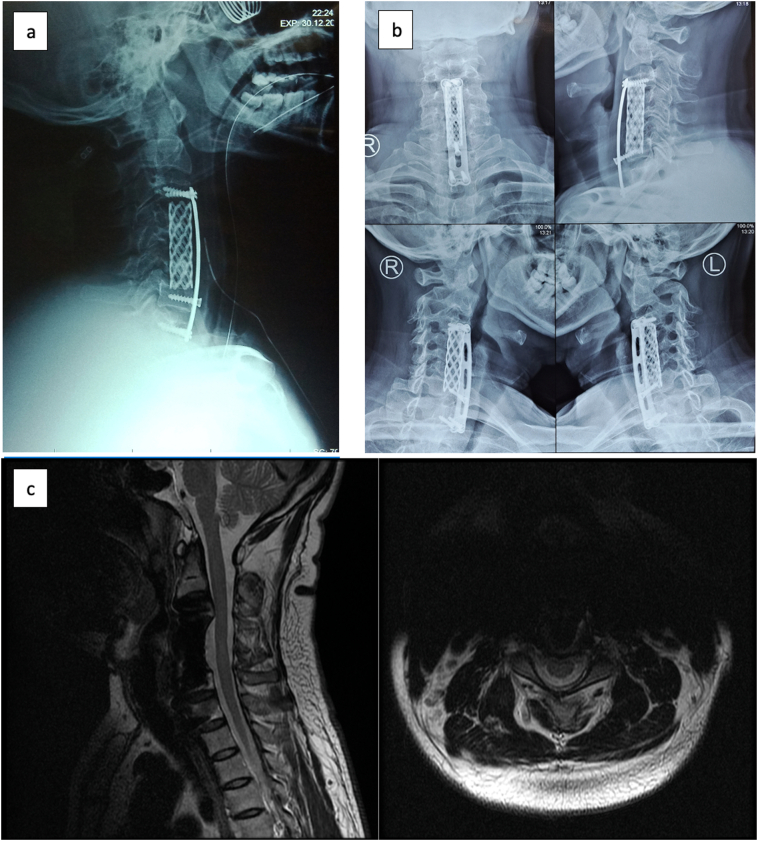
(a) Cervical X-Ray early post-operative; (b) 1-year post-operative follow-up Cervical X-ray; (c) 1-year post-operative follow-up Cervical MRI.
